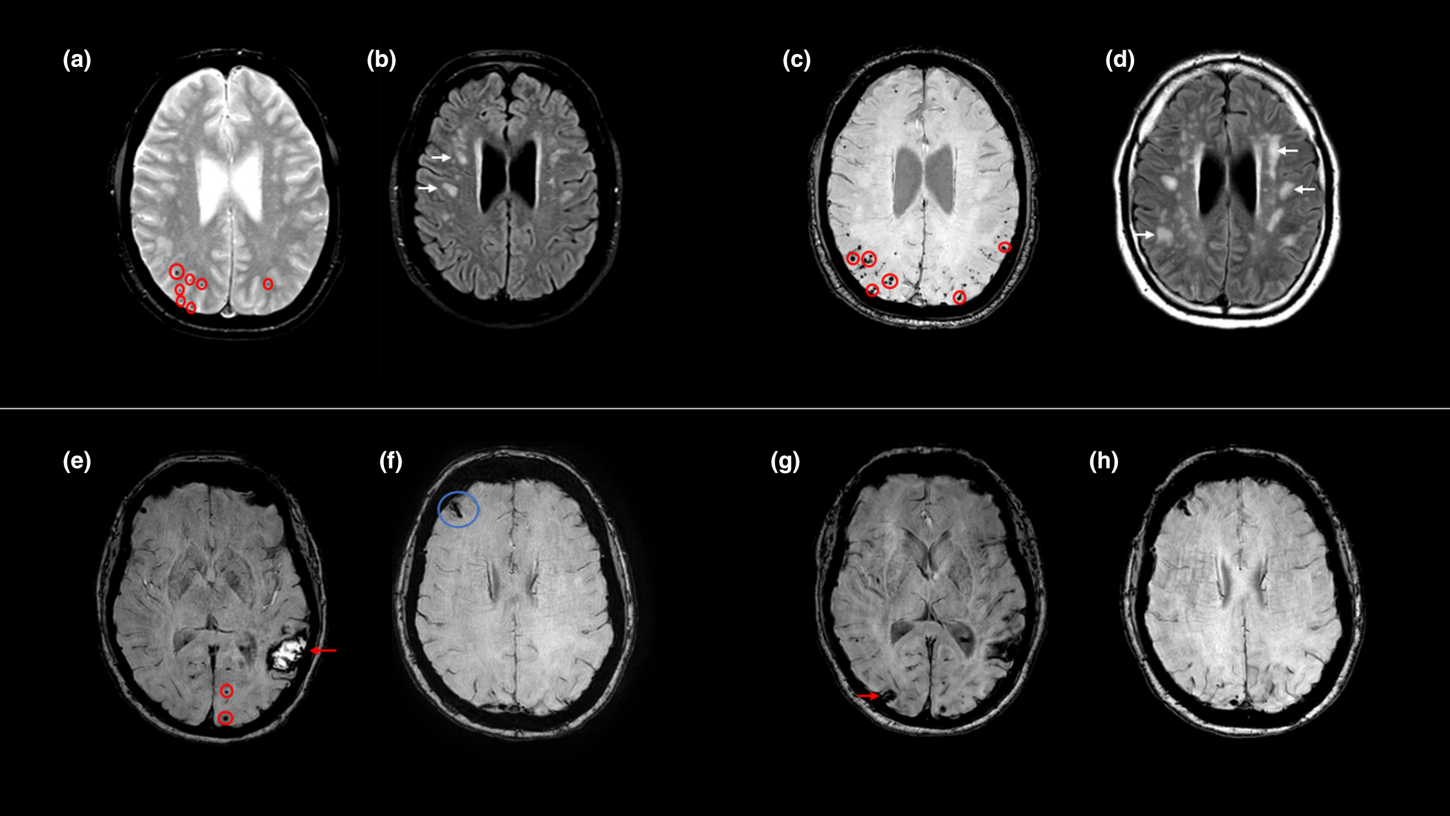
Radiological findings of both cases. Case 1: First MRI (1.5 T) showed >20 cerebral microbleeds (CMBs) ((a) red circles; T2 hemo‐sensitive), predominantly in the parietal, occipital and temporal lobes. There were focal white matter hyperintensities (WMHs) ((b) white arrows; T2 fluid attenuated inversion recovery, FLAIR) with beginning confluence and enlarged perivascular spaces in the centrum semi‐ovale (CSO‐EPVS; not shown; 21–40 visible; T2 turbo spin echo), in the absence of cortical superficial siderosis (cSS), previous ICH or deep hemorrhagic markers. Follow‐up MRI (3 T) after 4 years showed >100 CMBs ((c) susceptibility weighted imaging, SWI) and multispot pattern WMHs ((d) FLAIR). Case 2: First MRI and follow‐up after 2 months (performed due to temporary hemiparesis and hemihypesthesia) showed a recent left temporal ICH ((e) red arrow; SWI) with multiple lobar CMBs (red circles), right frontal focal cSS ((f) blue circle), multiple WMH spots and CSO‐EPVS (1–10 visible; both not shown). Repeat MRI 1 month later, performed due to headache and impaired vision, showed a new right parietal ICH and multispot pattern WMH (not shown). MRI (3 T) at 9 months also showed this ICH (g) and a new left parietal ICH (not shown) without further progression of cSS (h), WMHs or CSO‐EPVS.
